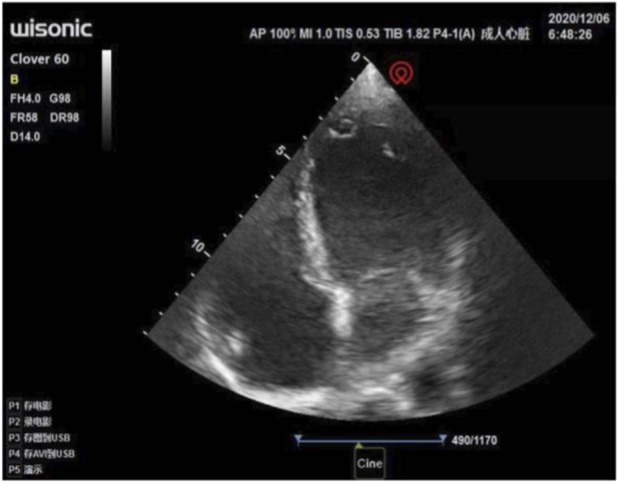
Transthoracic echocardiography shows segmental wall motion abnormalities, with decreased systolic activity in the anterior septum and the basal to apical segments of the anterior wall of the left ventricle.
